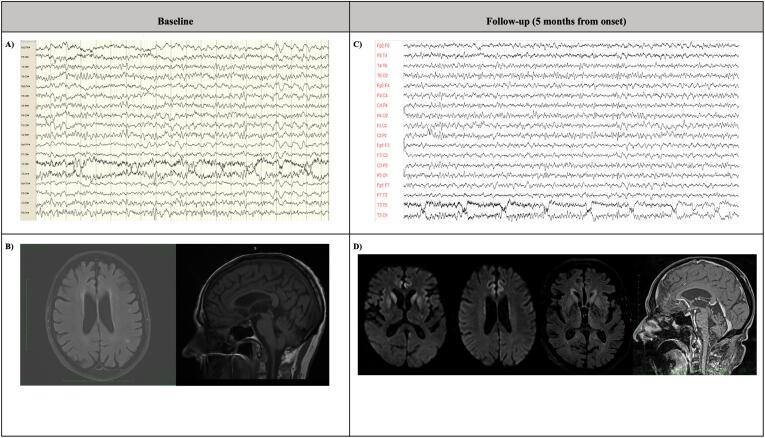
Video-eeg and brain imaging tests at baseline and at follow-up (five-months from the onset). A) EEG at baseline: it showed non-specific bilateral centrotemporal theta activity. B) Brain MRI (from left to right FLAIR and T1 sequence are displayed) at baseline: it showed chronic vascular encephalopathy; no hummingbird sign in sagittal T1-sequence. C) Video-EEG at follow-up: it evidences non-specific diffused theta and delta patterns with a typical repetitive pattern of bilateral synchronous quasi-periodic triphasic waves localized on the posterior regions of the brain. D) Brain MRI at follow-up (from left to right DWI, FLAIR and T1 sequences are displayed) demonstrating DWI hyperintensity (bright areas) in the caudate and putamen (hockey-racket sign) and the cortical ribbon. Abnormal hyperintensity is also present on the FLAIR sequence. No hummingbird sign in sagittal T1-sequence. Abbreviations:EEG, electroencephalogram; MRI, magnetic resonance imaging.
